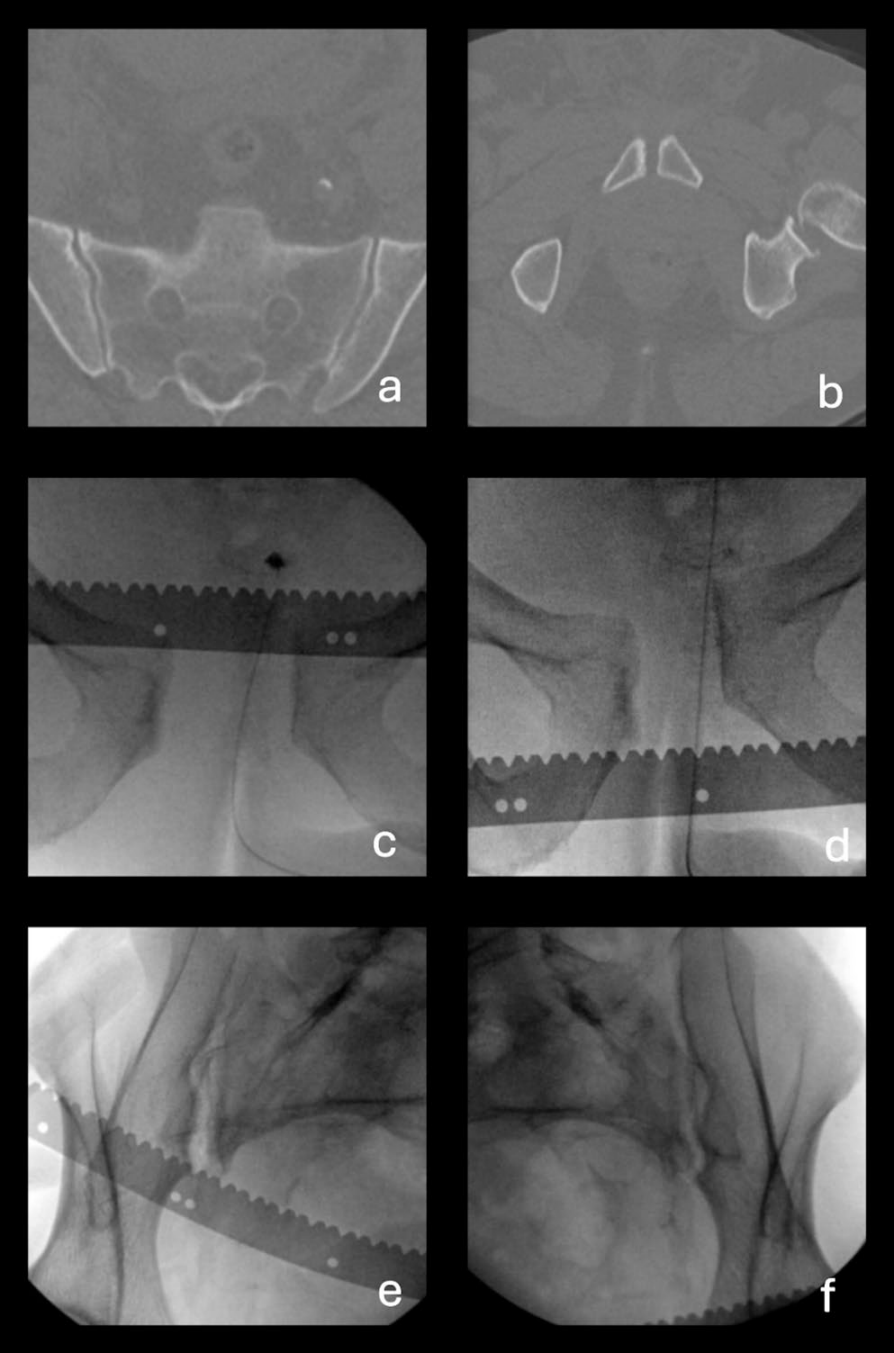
Case 9, which is an example of the classification changing from anterior-posterior compression 1 (APC1) to anterior-posterior compression 2 (APC2), with the added value of observing the sacroiliac (SI) joint being the ability to determine which side the diastasis is located on. (a) axial CT scan of the sacrum with a pelvic binder on, showing no apparent SI diastasis (b) axial CT image of pubic symphysis showing minimal symphyseal diastasis (c) Anterior-posterior (AP) examination under anaesthesia (EUA) image of pubic symphysis in the external rotation stress maneuver, showing 4 cm of diastasis, but providing no insight into which SI joint is affected (d) AP EUA image of pubic symphysis in the push/pull stress maneuver, showing 1.1 cm of rotational instability, but providing no insight into which SI joint is affected (e) Obturator inlet EUA image of right SI in the external rotation stress maneuver, demonstrating diastasis in the anterior SI joint (f) Obturator inlet EUA image of left SI in the external rotation stress maneuver, showing no SI diastasis
